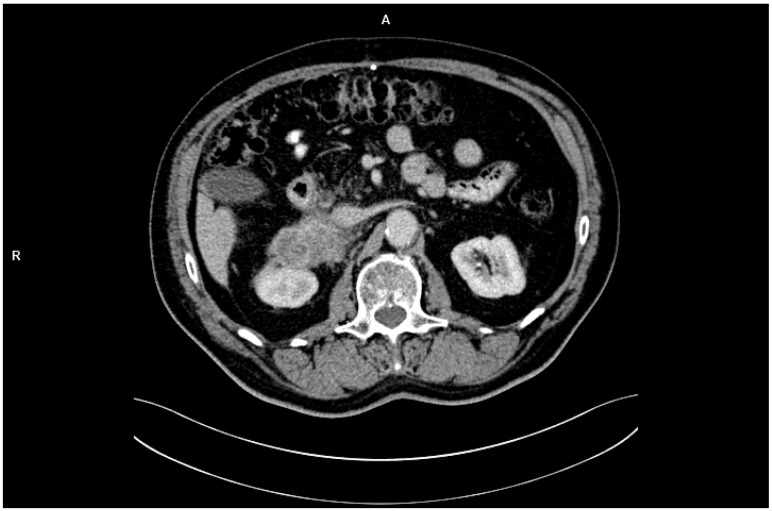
CT imaging with identified a 7 × 9 cm mass in the right adrenal gland.
